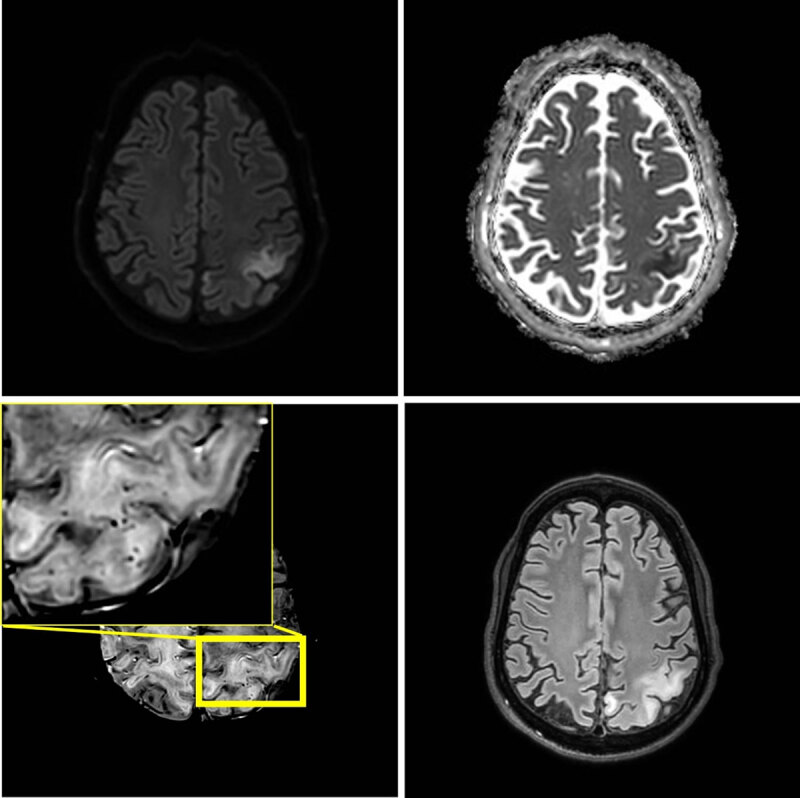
One month later, seven weeks after the initial episode, a third stroke-like event occurred. MRI shows a new area of edema in the left parietal lobe including the postcentral gyrus. The new lesion shows diffusion restriction and juxtacortical microhemorrhages.
